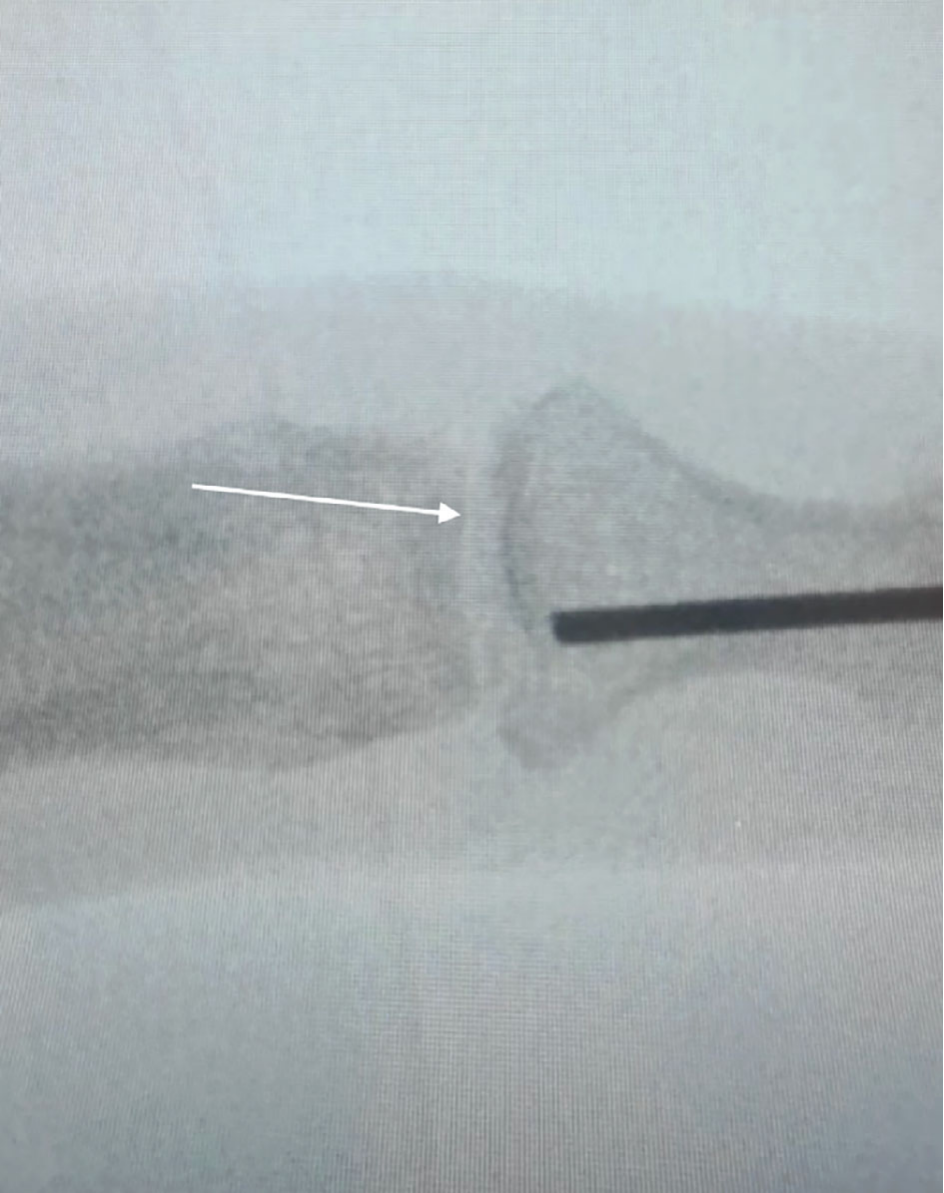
Intraoperative radiograph showing successful indirect reduction of DIP joint (white arrow) using the K‐wire as a bone tamp.
